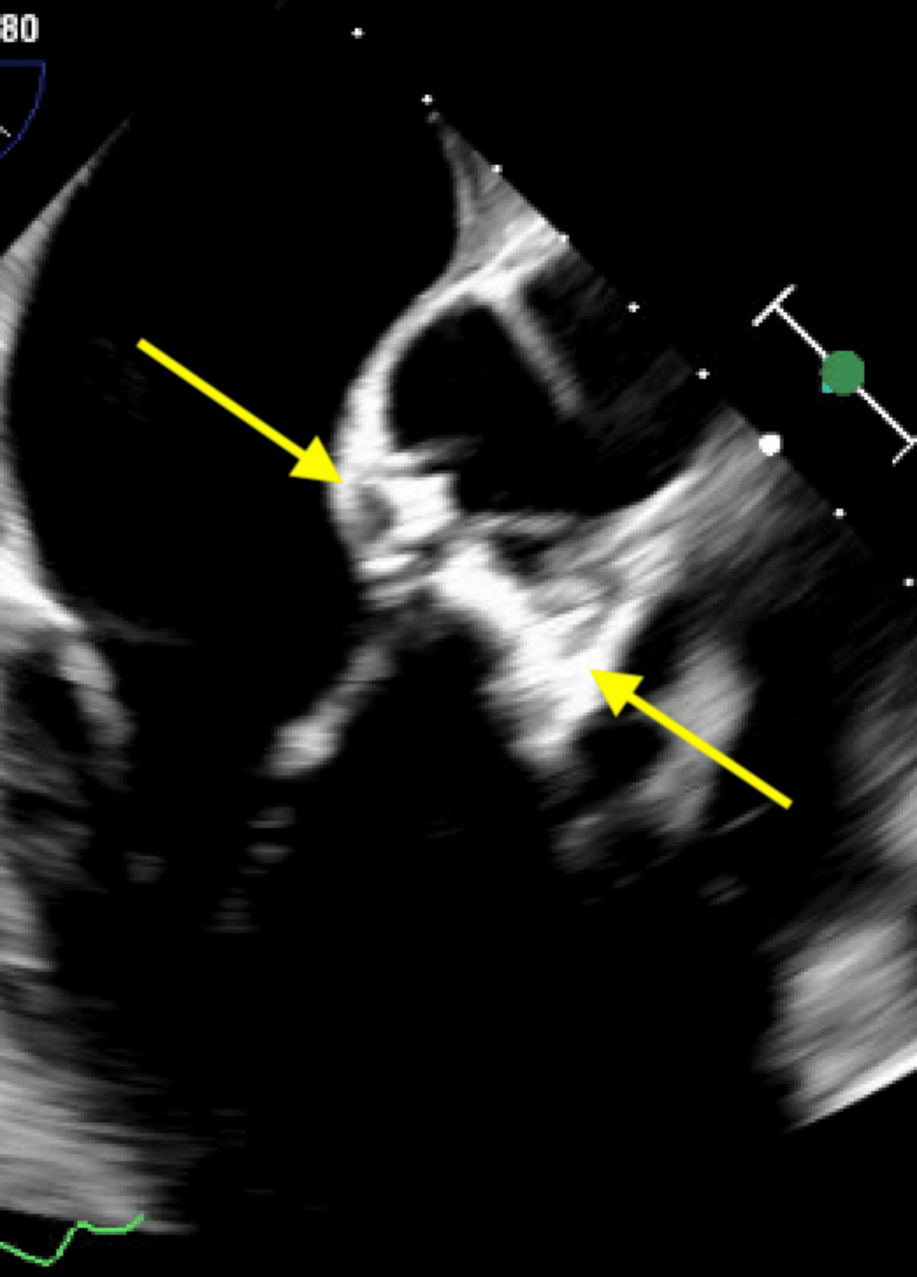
Transesophageal echocardiogram demonstrating an echodense, shaggy appearance of the aortic root, suggestive of an aortic root abscess (yellow arrow).
